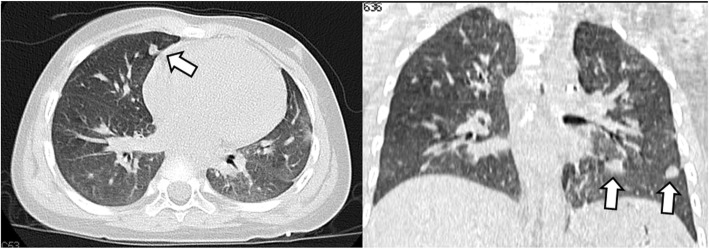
Computed tomography image of the chest showing multiple lung nodules (*arrows*)
